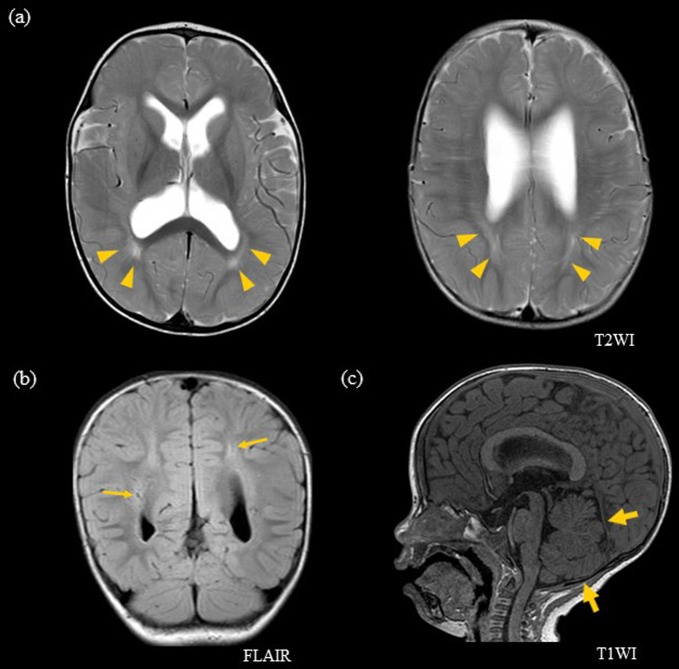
Head MRI at 10 months of age. a Axial T2-weighted image and b coronal FLAIR image show hyperintensity in the bilateral periventricular white matter regions (yellow arrowhead) and enlarged periventricular spaces (thin yellow arrow). c Sagittal T1-weighted image shows a narrow posterior fossa (broad yellow arrow)
